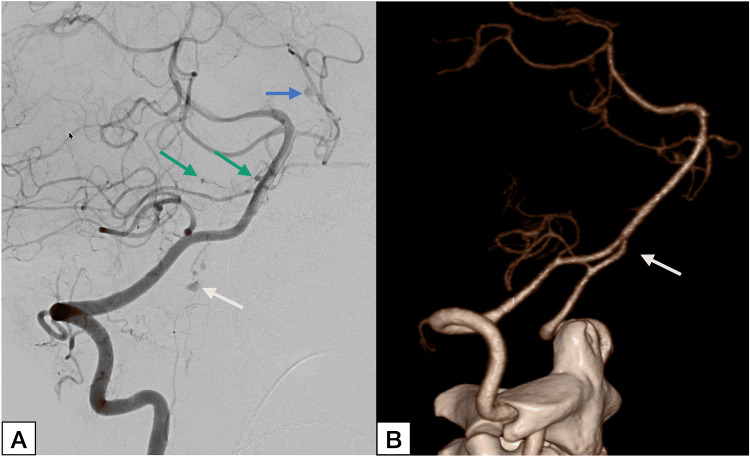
Detection of small DADA2-associated intracranial aneurysms using digital subtraction angiography versus computed tomography angiography (A) Oblique right vertebral artery digital subtraction angiography demonstrating multiple small aneurysms of basilar perforator arteries (green arrows), the left superior cerebellar artery (blue arrow), and the superior segment of the anterior spinal artery (white arrow). The multiplicity, small size, and involvement of distal posterior circulation and spinal vessels are atypical for sporadic saccular aneurysms and suggest an underlying inflammatory arteriopathy. (B) Three-dimensional reconstruction of computed tomography angiography of the same vascular territory demonstrating no detectable aneurysms; the anterior spinal artery aneurysm (white arrow) is not visualized.
